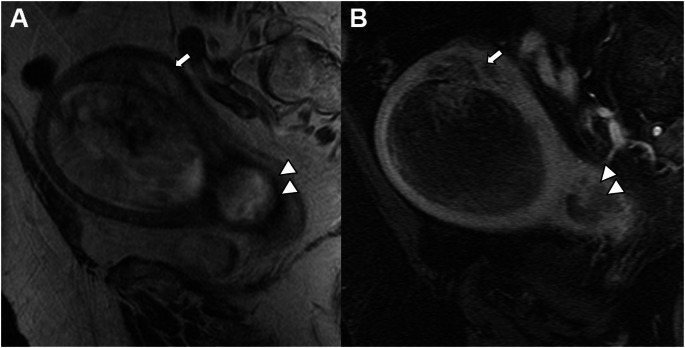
Stage IIA grade 2 endometrioid carcinoma, no LVSI, in a 65-year-old woman. A Sagittal T2-weighted fast spin-echo (FSE) image (TR/TE: 4500/100 ms; slice thickness: 3 mm) and B sagittal contrast-enhanced 3D T1-weighted fat-suppressed LAVA sequence (TR/TE: 4.2/2.1 ms; slice thickness: 2 mm; post-gadolinium, delayed phase at 4 min) show disruption of the junctional zone (arrow). The tumor extends more than 50% into the myometrium and into the cervical canal with disruption of the cervical stroma (arrowheads), best seen on delayed phase images
